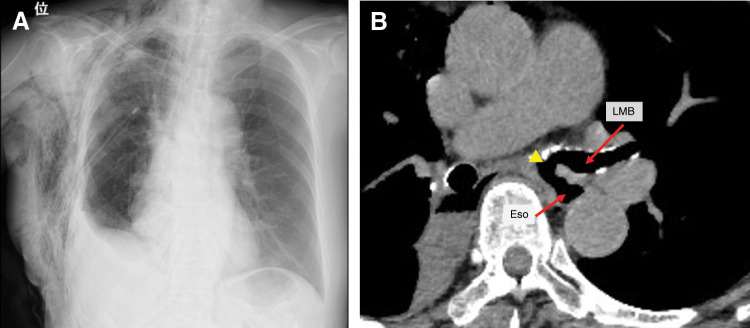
Chest radiograph and CT images at readmission. (A) Chest radiography revealed mild collapse of the right lung and subcutaneous emphysema. (B) Axial contrast-enhanced CT image at the subcarinal level demonstrating a suspected communication (yellow arrowhead) between the LMB and the Eso. The LMB and the Eso are indicated by red arrows. Eso, esophagus; LMB, left main bronchus
